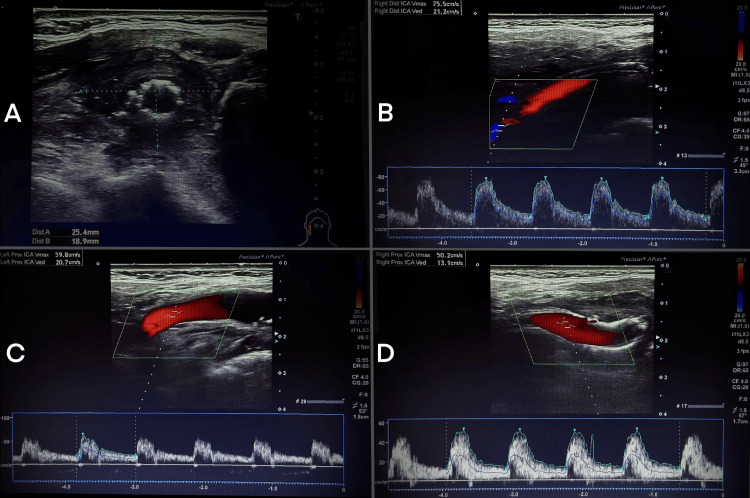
Postoperative ultrasound findings: (A) B-mode image visualizing the excluded PsA and a well-deployed stent, (B-D) triplex ultrasound (B-mode, color Doppler, and pulsed-wave Doppler) demonstrating distal flow velocity in the right ICA (B), proximal flow velocity in the left ICA (C), and proximal flow velocity in the right ICA (D). PsA: arterial pseudoaneurysm, ICA: internal carotid artery
